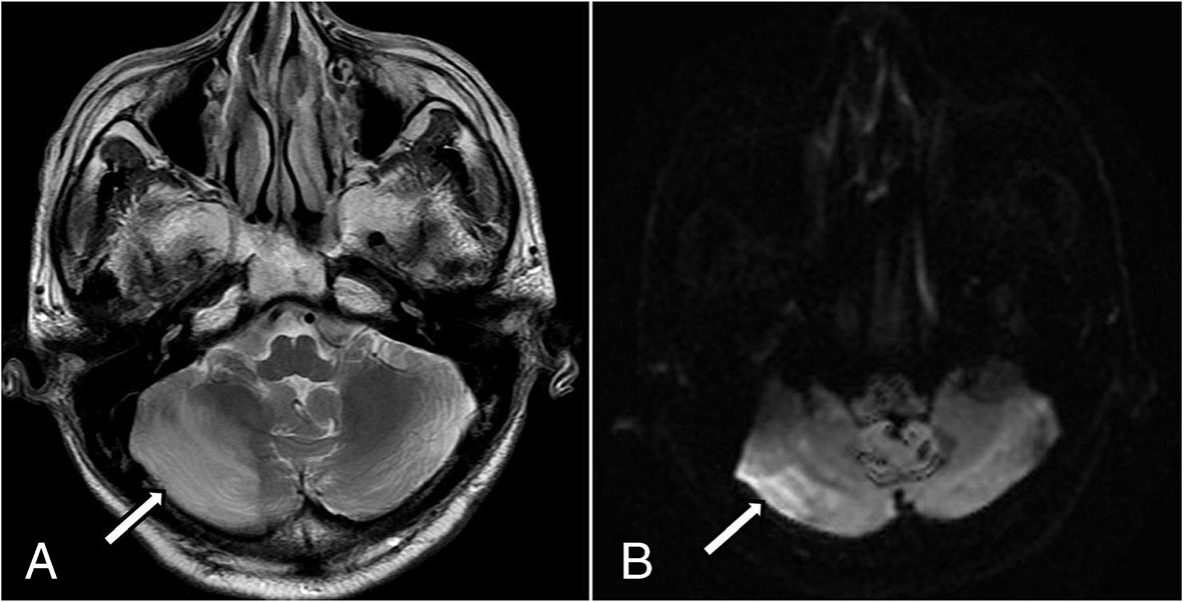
(A) Axial T2-weighted magnetic resonance image shows diffuse high signal intensity in the right cerebellum (arrow). (B) Diffusion-weighted magnetic resonance image at the same level demonstrates an increased diffusion restriction in the same region (arrow).
